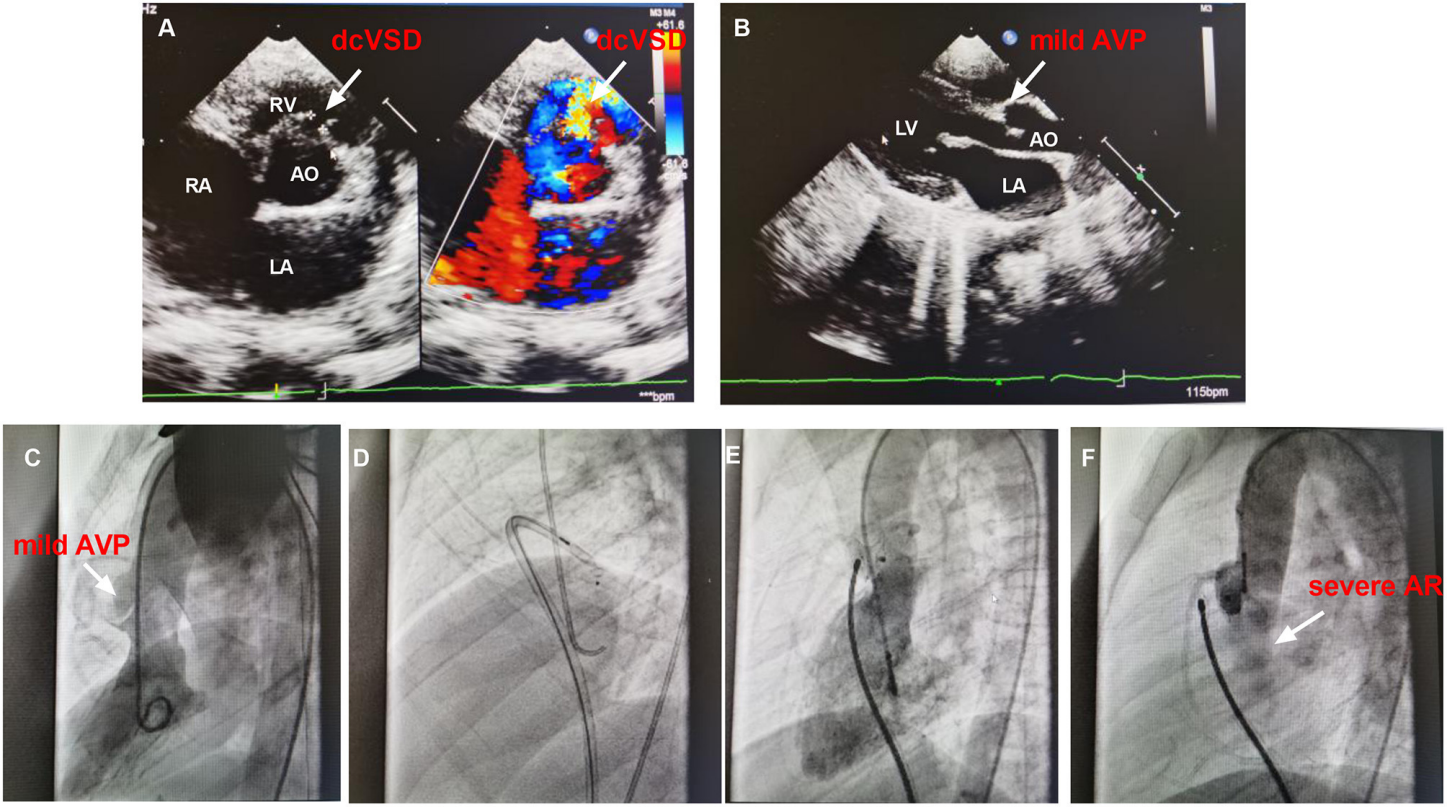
The images of TTE and angiography of case 12. (A,B) shows the dcVSD and the mild AVP on TTE. (C) Shows the mild AVP by left ventricular angiography. (D) Demonstrates the closure using ADO-II and (E) Shows the closure with symmetrical double-disk occluder. (F) Shows the AR after deploying occluder. AO, aorta; AR, aortic regurgitation; AVP, aortic valve prolapse; dcVSD, doubly committed subarterial ventricular septal defect; LA, left atrium; LV, left ventricle; RA, right atrium; RV, right ventricle; TTE, transthoracic echocardiography.
